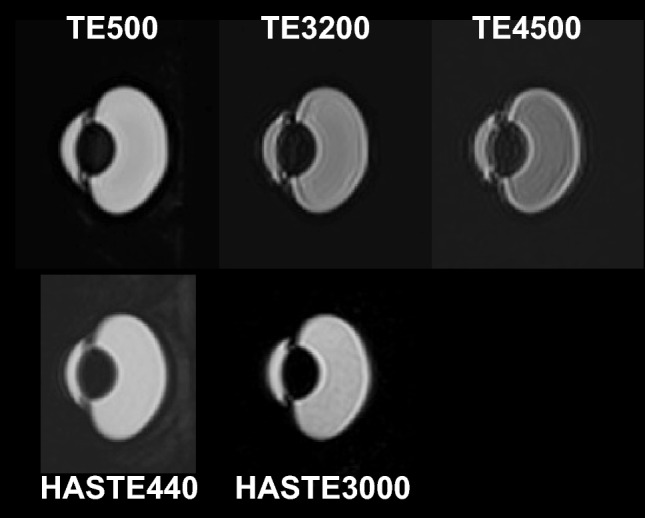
Sample images with 3D T2-weighted and HASTE sequences before topical eye drop administration. Ultra-heavily T2-weighted sequences (TE3200 and TE4500) had blurring artifacts, whereas the HASTE sequence with super long TE (HASTE3000) provided artifact-free images
